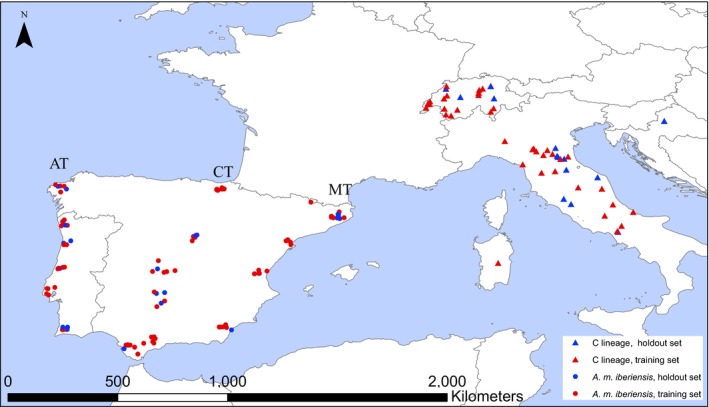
Geographic locations of the 176 whole‐genome sequenced individuals. The Iberian honeybees are distributed across the three transects: Atlantic (AT;* N* = 31), Central (CT;* N* = 61) and Mediterranean (MT,* N* = 25). Each dot represents a single colony and apiary
